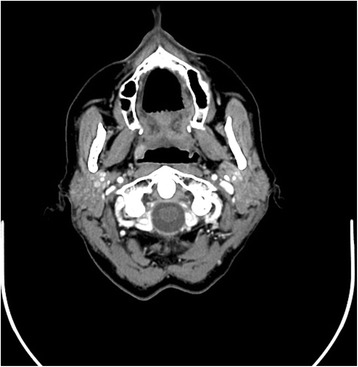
This image shows a contrast CT scan of the neck, axial view, demonstrating a suspicious mass in the lateral right soft palate
